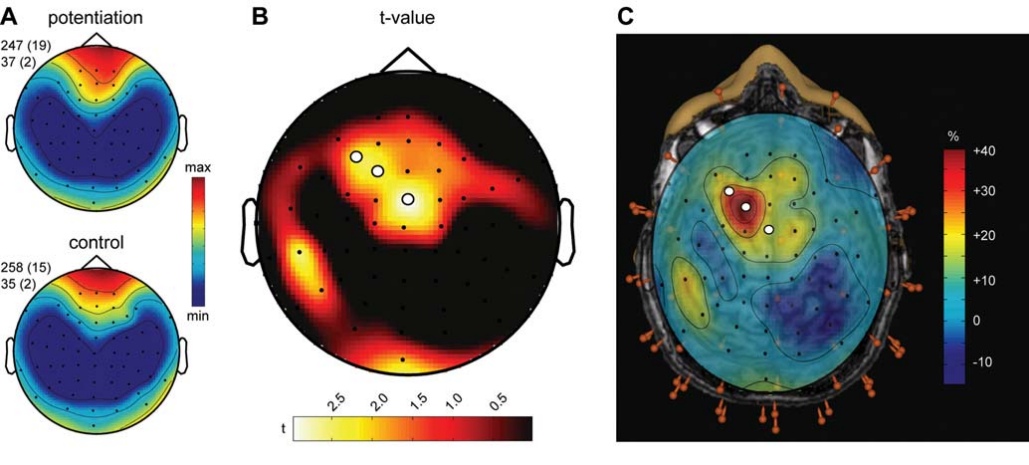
Changes in local SWA homeostasis during sleep after 5-Hz rTMS conditioning. A. Topographic distribution of SWA after 5-Hz conditioning (top) and the sham control (bottom) condition. Average EEG power density at 1–4.5 Hz (n = 10 subjects) for the first 30 minutes of NREM sleep. Values were normalized by total power for the recording, color coded, plotted at the corresponding position on the planar projection of the scalp surface, and interpolated (biharmonic spline) between electrodes (dots). Values to the left of the topographic plots represent maximal and minimal power (in percentage of the overall mean) with standard errors in parenthesis. B. Topographic distribution of the t-value for the comparison between the 5-Hz conditioning and sham control condition (two-tailed paired t-test). White dots indicate electrodes showing significant differences after statistical non-parametric mapping (see methods). C. Anatomical localization of the three electrodes showing a significant difference in SWA during the first 30 min of NREM. All 60 electrodes (red pins) were digitized and co-registered with the subject's magnetic resonance images. When the topographic distribution of the percentage change of SWA after the TMS conditioning compared to the control condition was projected onto the brain, the three significant electrodes projected onto left premotor cortex (white dots).
